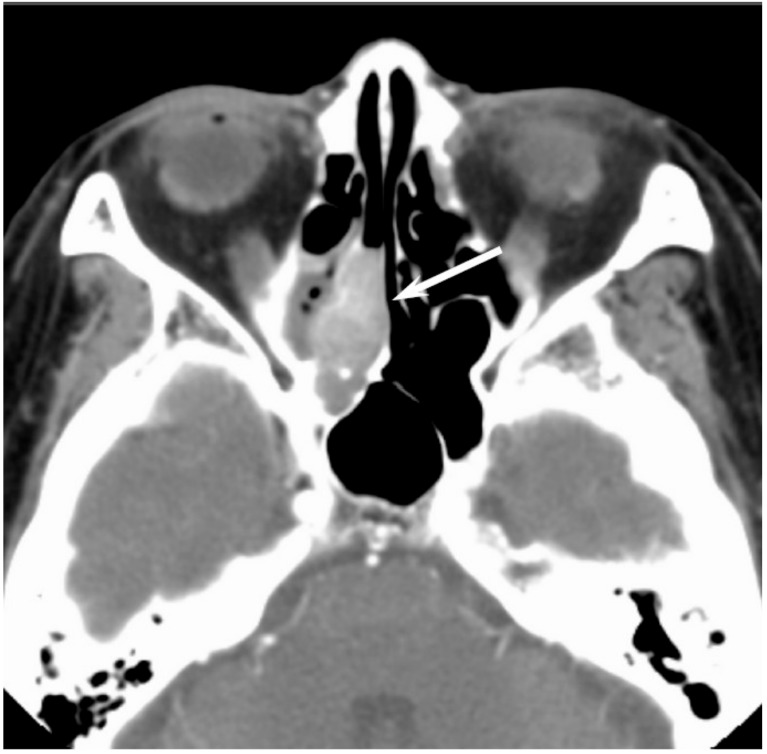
Olfactory neuroblastoma of the right nasal cavity. Contrast-enhanced CT image showing a homogeneously enhanced lesion in the right olfactory cleft (arrow).
